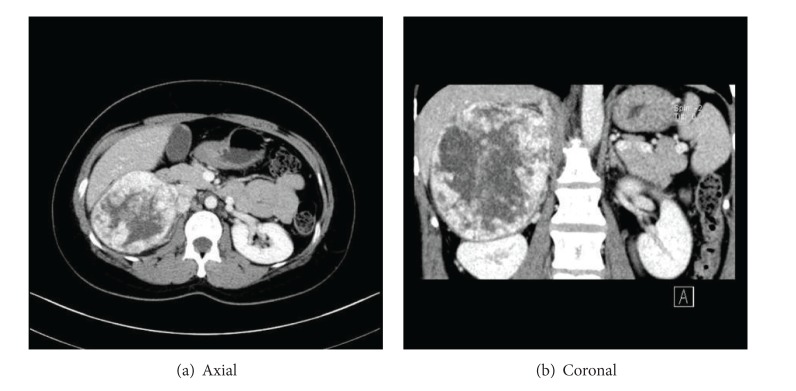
CT scan of the abdomen showing a mass in the right adrenal gland.
